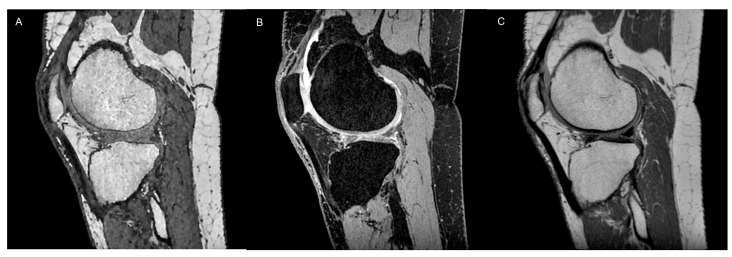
Cartilage morphometry of a 51-year-old male through 3D sequences, which allow quantitative assessment of cartilage morphological characteristics, like a T1-weighted spoiled gradient echo (SPGR, A), a 3D GRE DESS sequence (B) namely MENSA (Multi-Echo iN Steady-state Acquisition), and a 3D proton-density CUBE sequence (C).
